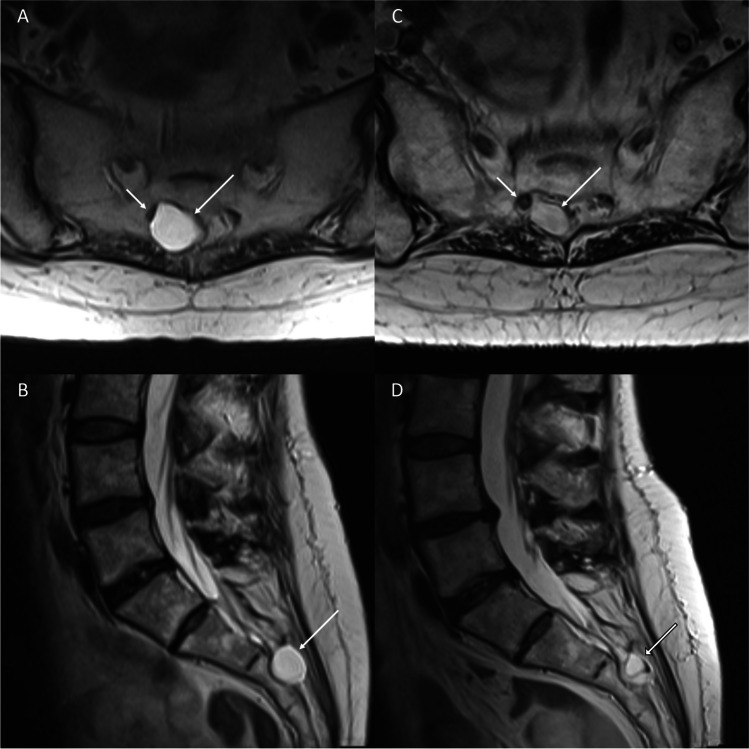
T2-weighted axial and sagittal images of a symptomatic right S3 Tarlov cyst belonging to the patient in Fig. 9, before and after fibrin sealant injection. A, B Preprocedural T2-weighted images show a large Tarlov cyst in the remodelled right S3 foramen (arrow) which compresses the right S3 nerve (short arrow). C, D Follow-up imaging performed at 32 months demonstrates lasting collapse of the cyst (arrow) with resolution of neural compression of the exiting S3 nerve which assumes a rounded morphology (short arrow)
